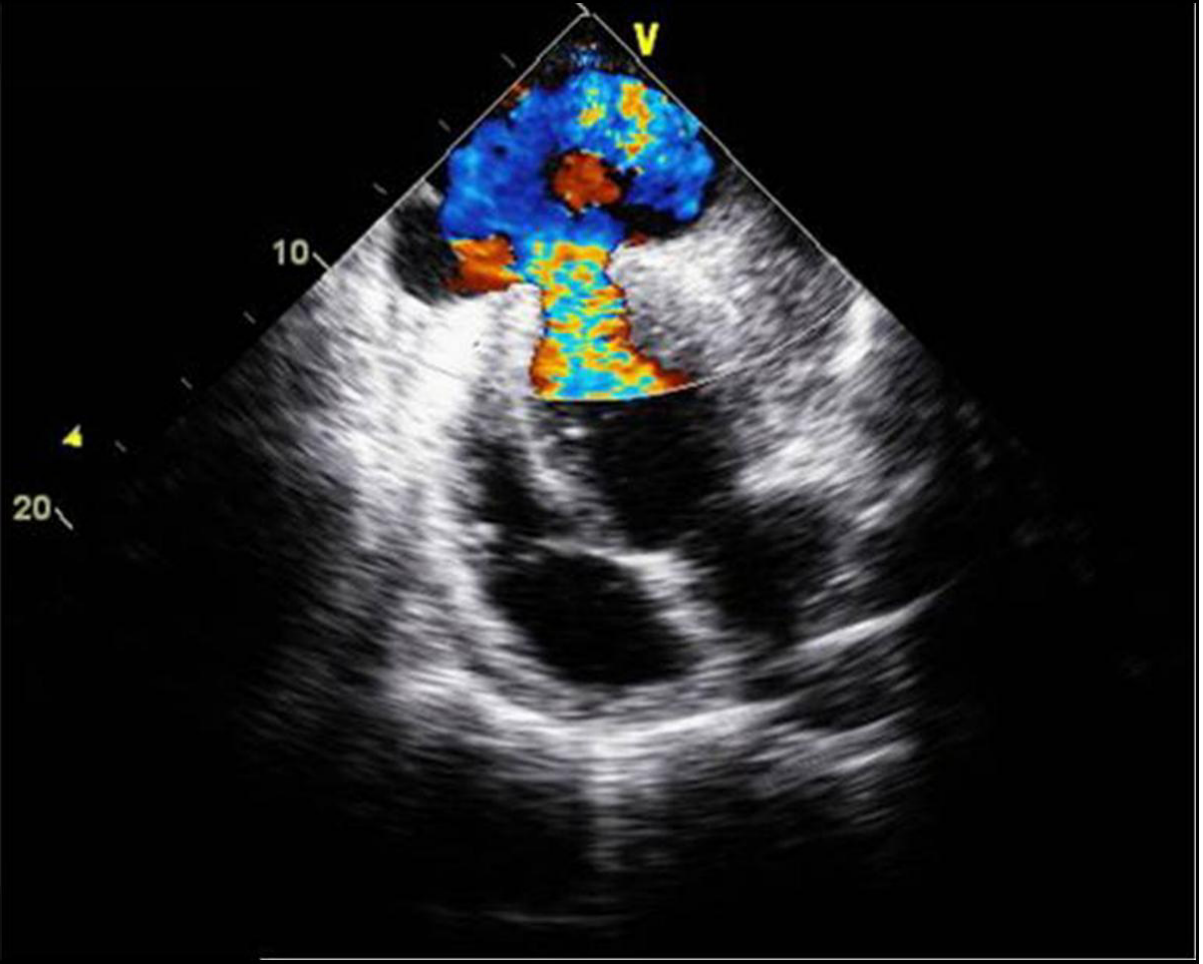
Transthoracic echocardiography of the apical pseudoaneurysm. Four-chamber view demonstrates a large discontinuity of the ventricular wall in the apex, with the image resembling a "mushroom cloud".
